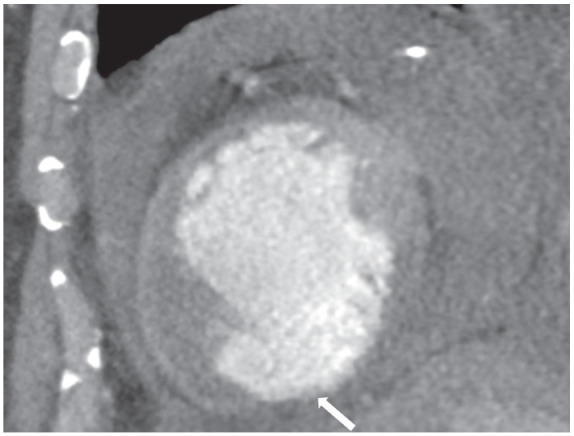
Short axis view of coronary computed tomography angiography study with contrast. Arrow indicates broad based aneurysm in the left ventricle.
